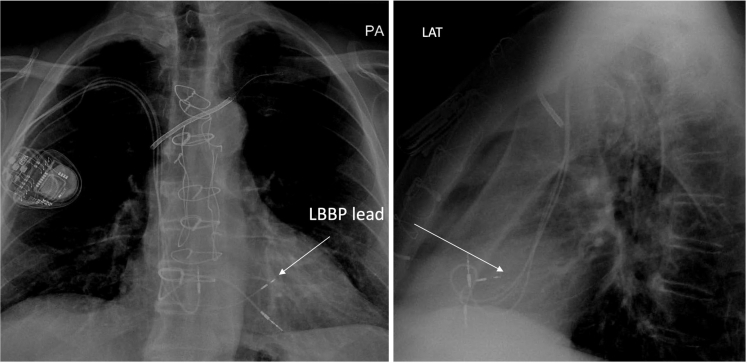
Posteroanterior and Lateral Chest X-Rays of the Left Bundle Branch Pacing Lead Location (Arrow)
